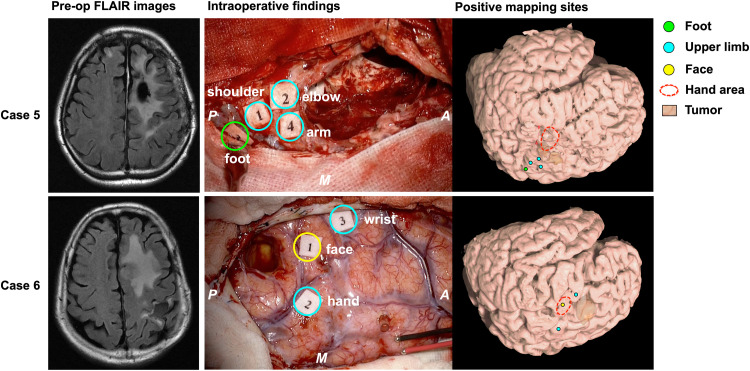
Patients whose motor function moved according to Type 2. The left columns show the preoperative FLAIR images; the middle columns show the intraoperative findings; the right columns show the positive mapping sites for each patient’s brain reconstructed from structural MRI. In Case 5 (upper column), the motor area of the upper limb was observed next to the foot area. In case 6 (lower column), the face area was found between the hand and the wrist area. Green circle: positive mapping site of foot; cyan circles: positive mapping sites of upper limb including shoulder, arm, elbow, hand, and wrist; yellow circle: positive mapping site of face; red broken line: knob area in the precentral gyrus, namely, the hand area. A: anterior; P: posterior; M: medial side.
